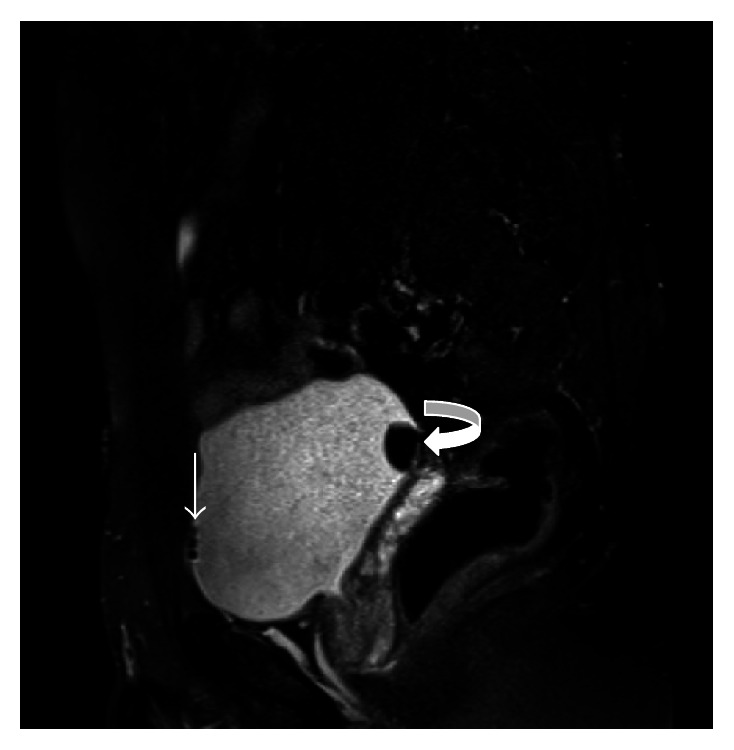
In MRI examination, there are hypointense air bubbles in anterosuperior part of bladder (arrow) and 2 cm diameter of calculi in posterosuperior part of bladder at T2-weighted image (curved arrow).
